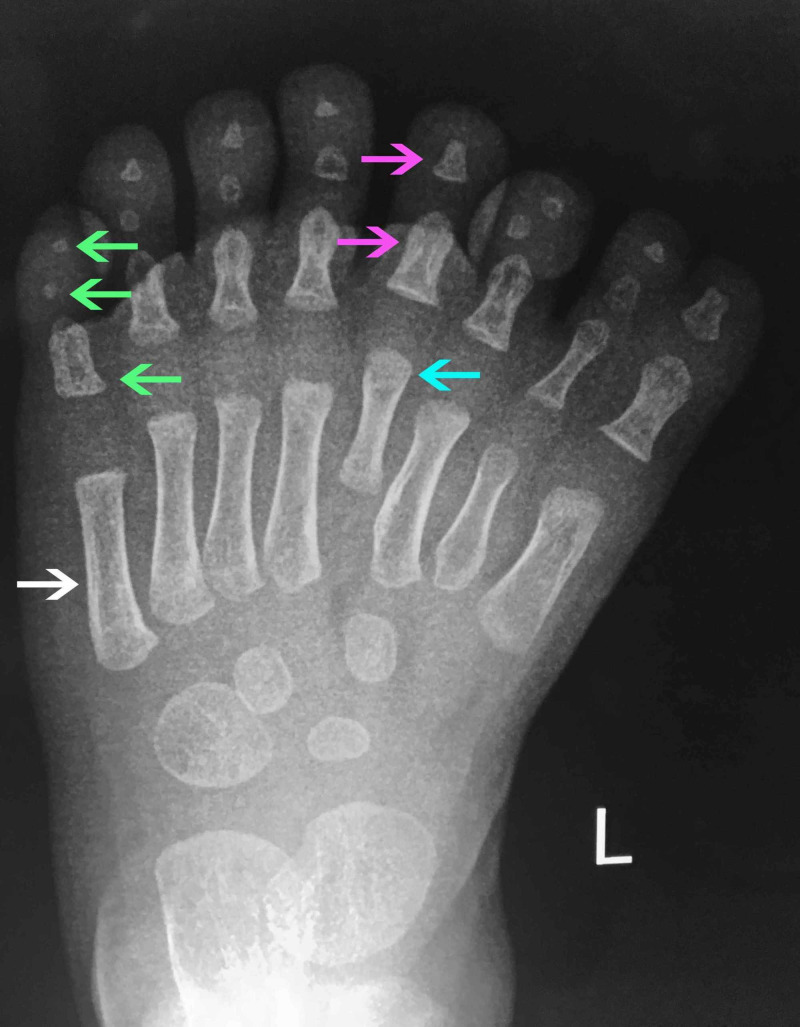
X-ray (XR) left central ray mirror foot XR left central ray mirror foot with eight digits portraying fully developed metatarsal (white arrow), proximal, middle, and distal phalanges (green arrows), except for the fourth toe from the medial side which appeared to be missing a middle phalanx (pink arrows) and had a dysplastic metatarsal (blue arrow), in comparison to the rest (white arrow). This digit was similar to a great toe.
